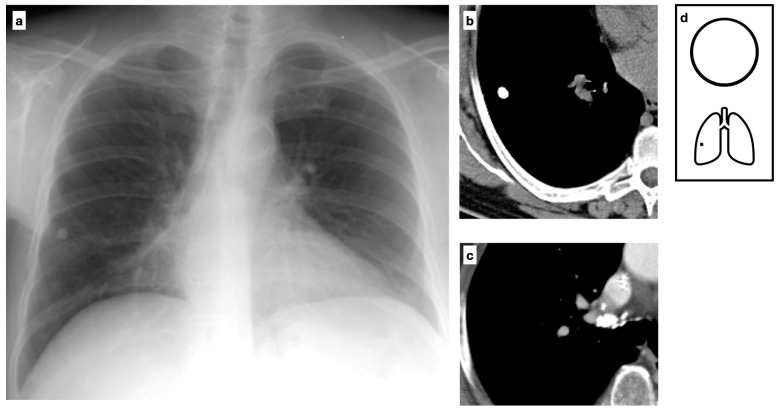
Chest X-ray shows a uniformly calcified lung nodule in the peripheral region of the right lower lobe (a). CT confirms the presence of a nodule with diffuse calcification, consistent with a Ghon focus, associated with ipsilateral calcific lymph nodes, forming the Ranke complex. The Ghon focus represents a site of primary tuberculosis with a fibrotic scar that may eventually calcify (b,c). Pattern of calcification (d).
